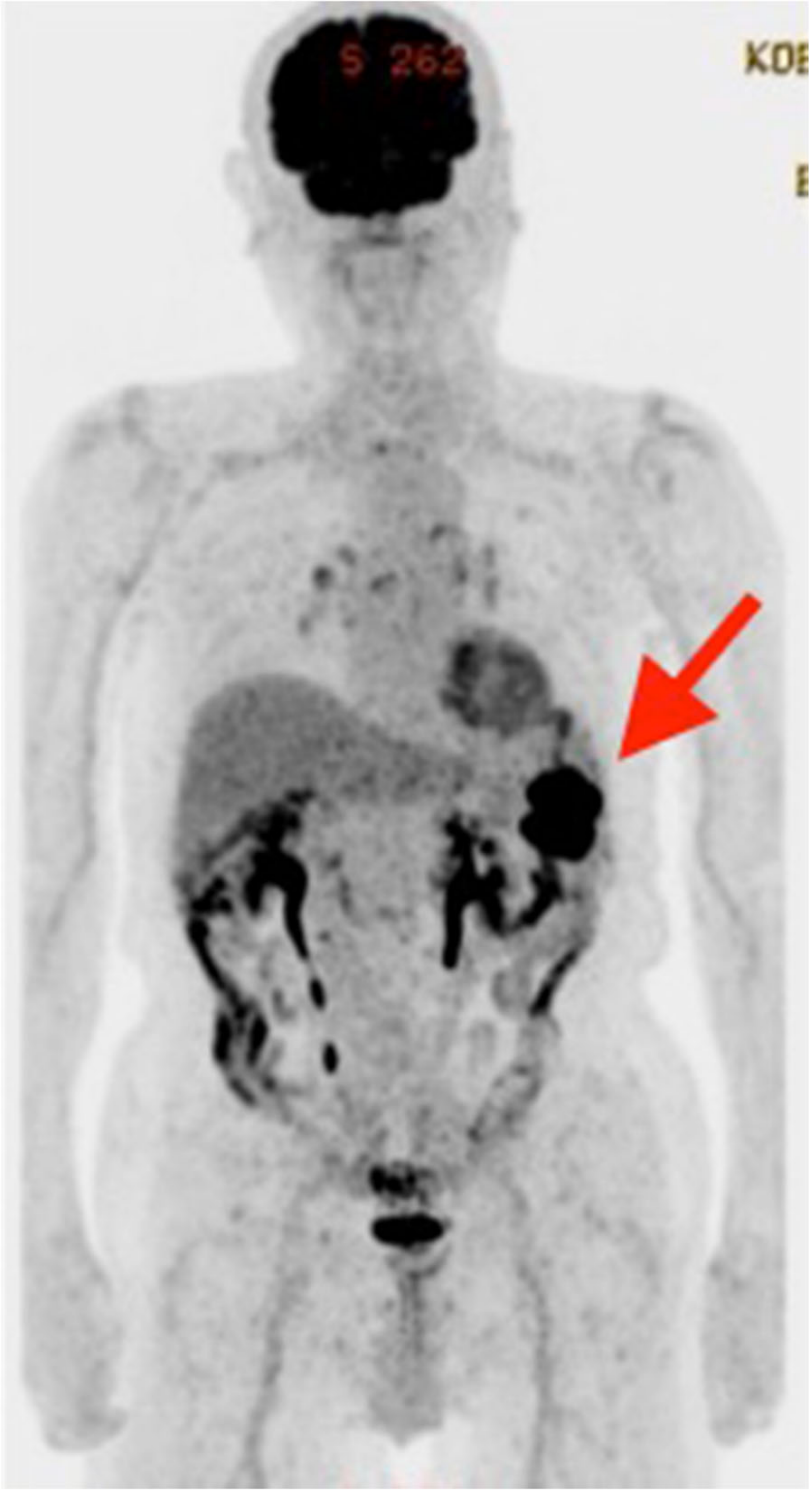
FDG-PET–CT showing abnormal FDG accumulation in the spleen (red arrow). FDG, ^18^F-2-deoxy-2-fluoro-glucose, PET–CT, positron emission tomography–computed tomography
